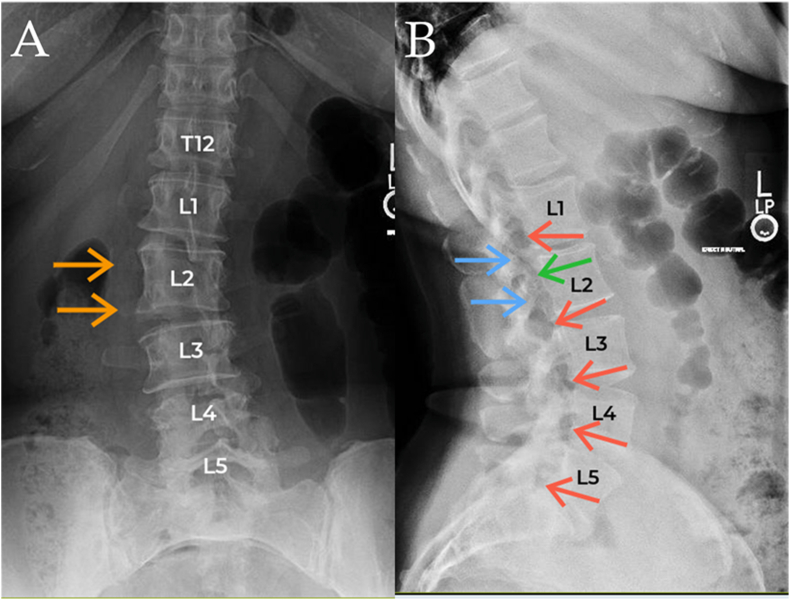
X-ray films of the thoracolumbar spine. The AP view (A) shows transitional anatomy as well as duplicate transverse process extending from the right L2 vertebral body (orange arrows). The lateral view (B) shows duplicate pedicles at the L2 vertebral body (blue arrows) as well as typically located intervertebral foramina (red arrows) and “pseudo foramen” located between the duplicate pedicles (green arrow). Note the “pseudo foramen's” slightly posterior location compared to other typical foramina. (For interpretation of the references to colour in this figure legend, the reader is referred to the Web version of this article.)
